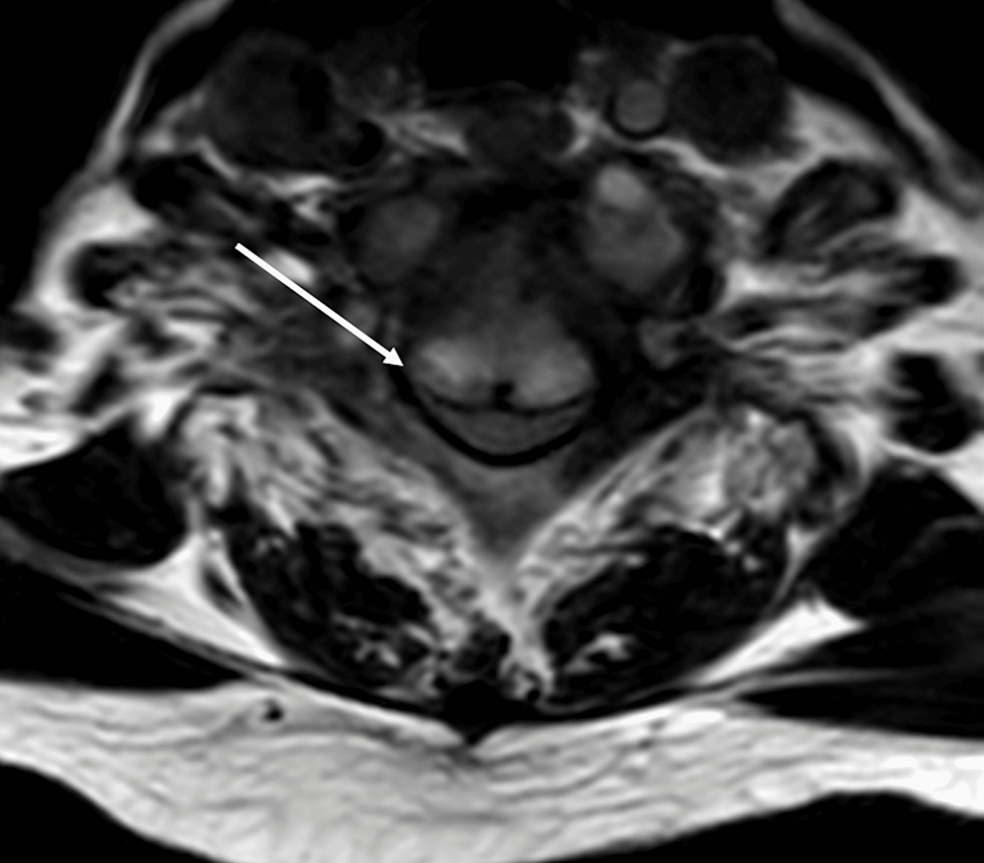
Axial cervical spine MRI portraying posterior repulsion of the C6/C7 vertebrae due to neoplasm versus infection
